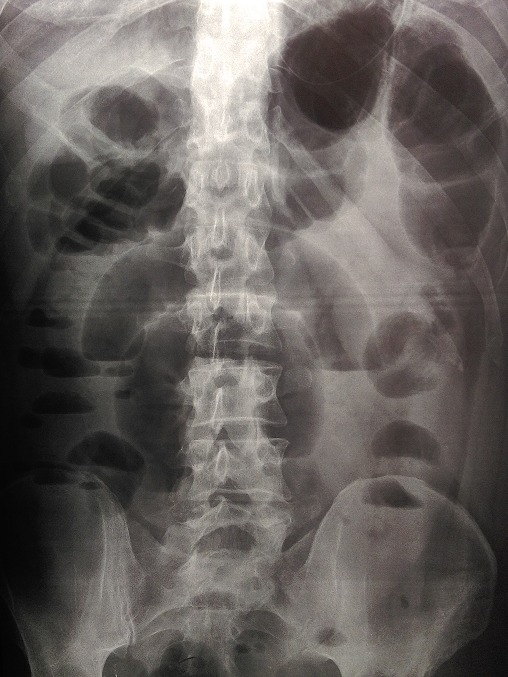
erect radiograph of the abdomen showing dilated small bowel loops with some air-fluid levels
